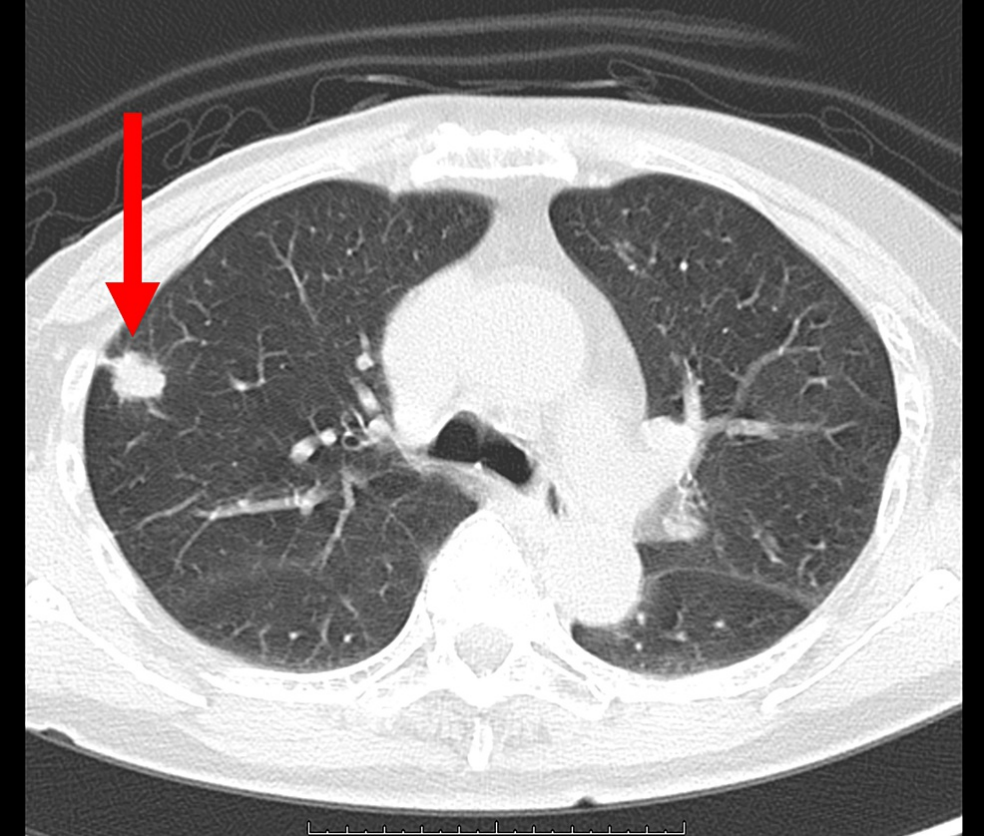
CT scan of the lung metastasis in case one A 16-mm long fine lobulated mass with spiculation can be observed in the S2b/3a region of the right upper lobe.
